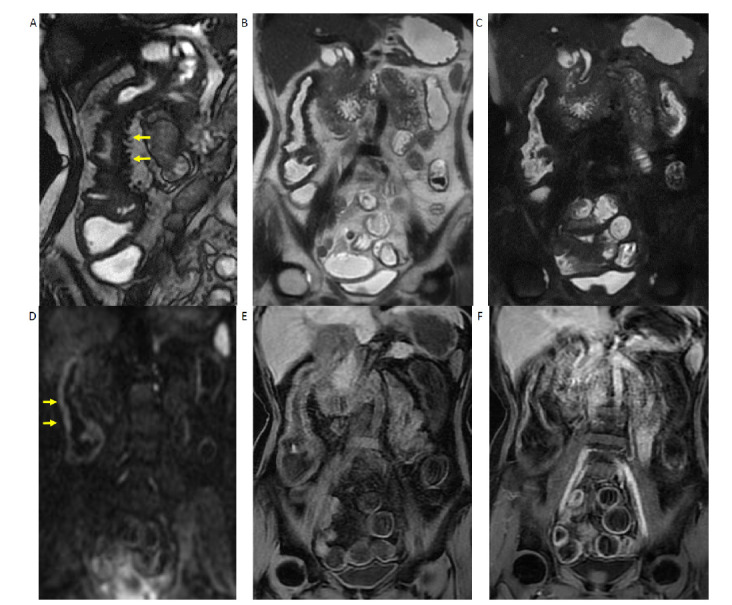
MR Enterography with 3% oral mannitol solution. (A) Fast imaging employing steady-state acquisition (FIESTA) sequence shows diffuse low signal intensity of the thickened ascending colon with multiple triangular signal voids along the mesenteric sides. (B) Fast spin-echo T2-weighted (FSE-T2W) and (C) fat-suppressed T2WI (FS-T2W) show no intramural edema and diffuse low signal intensity of ascending colon. Transverse colon is similarly affected; the terminal ileum is normal (not shown). (D) Diffusion-weighted images demonstrate moderate hyperintensity along the anti-mesenteric side of ascending colon. (E) Pre-contrast and (F) post-contrast T1-weighted images demonstrated no appreciable contrast enhancement at venous phase.
